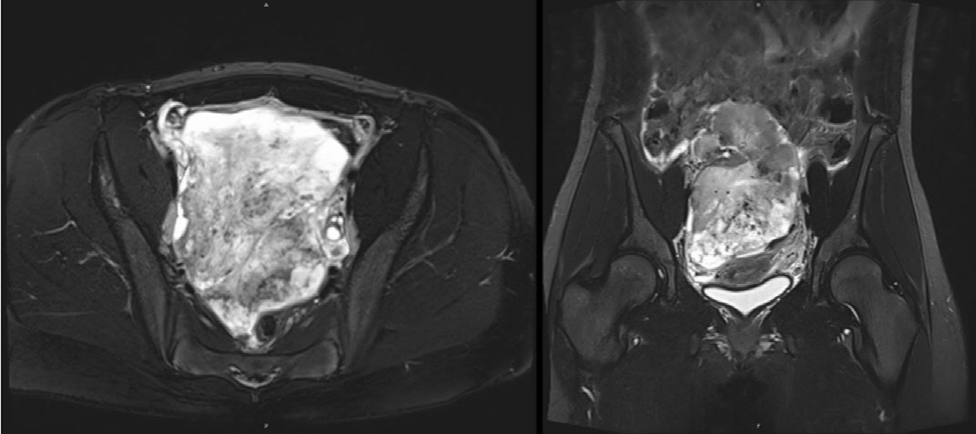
Preoperative MRI scan (T2-weighted sequence) revealed a large pelvic mass of unknown origin. MRI, magnetic resonance imaging.
